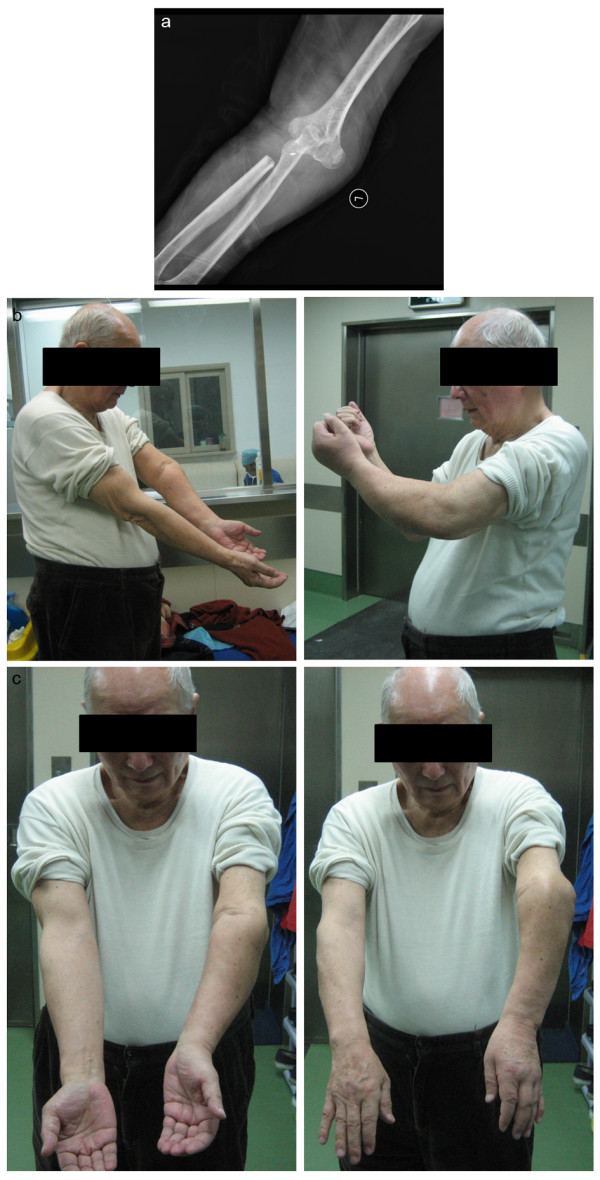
Postoperative results for left elbow showing: (a) postoperative radiograph at 6-month follow-up, (b) extension and flexion, and (c) pronation and supination.
